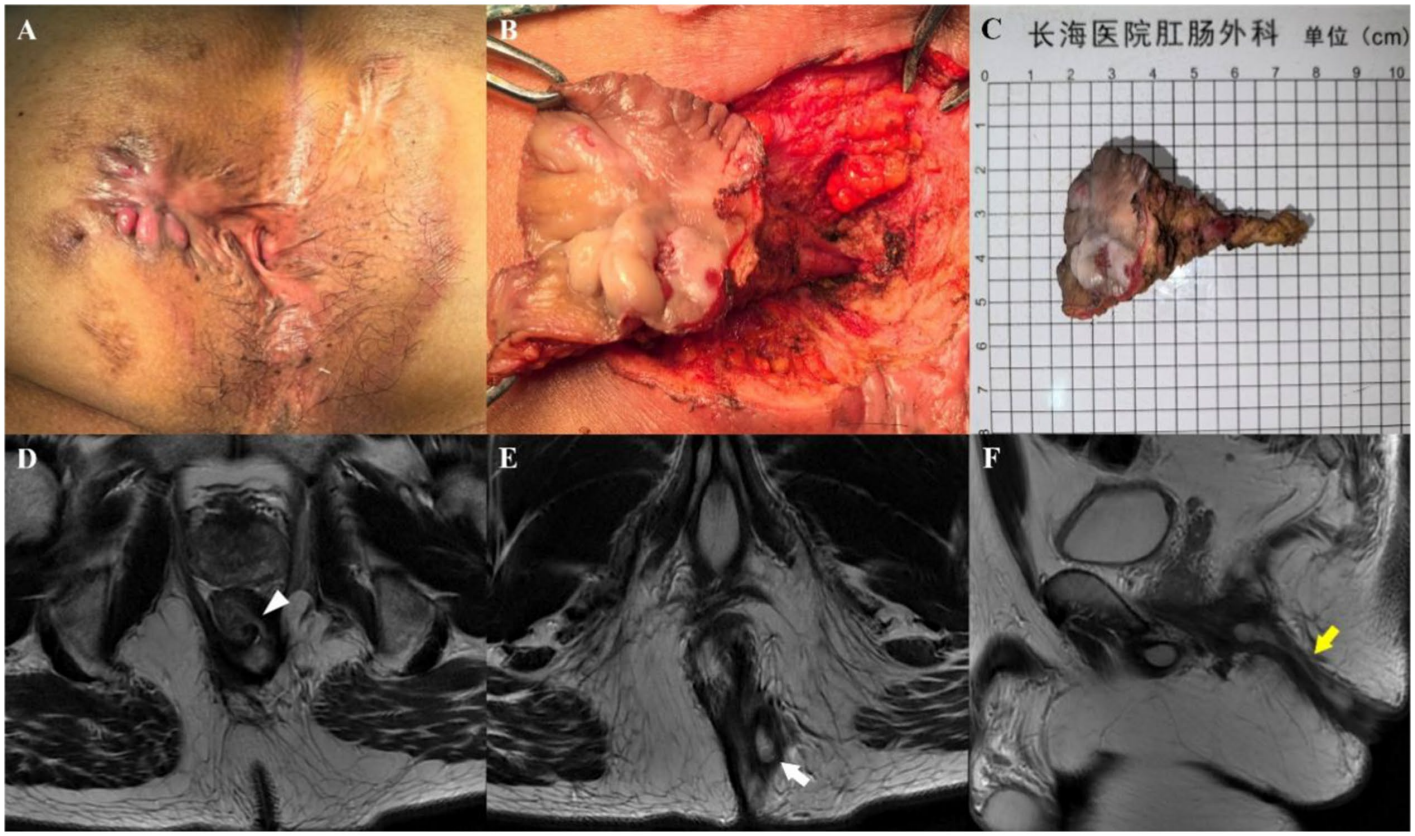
A 47-year-old man who underwent three anal fistula surgeries at another hospital. Preoperative appearance (A) and findings during the operation (B) showing a transsphincteric fistula. (C) Sample from surgical resection. Axial T2-weighted images without fat suppression show a left transsphincteric fistula crossing both layers of the sphincter complex, with an internal opening at the 3 o’clock position (D, arrowhead) and an external opening at the 5 o’clock position (E, arrow). Sagittal T2-weighted image without fat suppression (F) showing the transsphincteric extension of the fistula backward (yellow arrow). Preoperative MRI findings demonstrated complete concordance with intraoperative exploration.
